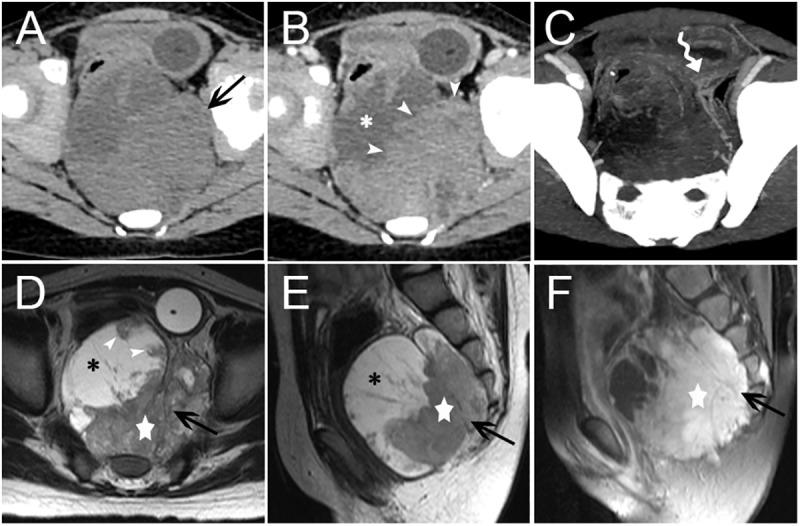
Presacral Region pPNET in a 5-year-old Female (Case no. 3). CT images showed an irregular, mixed solid cystic mass (arrow) with an indistinct margin in the presacral region (A). The necrotic or cystic portion (asterisk) did not show clear enhancement, but the solid portion (arrowheads) presented with intermediate heterogeneous enhancement (B). Blood supply arteries could be observed (curved tail arrow) (C). Non-contrast MRI images showed the solid components (five-pointed star) of the mass (arrow) was isointense on T1WI images and mildly, heterogeneous hyper-intense on T2WI images. The lateral necrotic or cystic portion (asterisk) showed hypo-intensity on T1WI images and heterogeneous hyper-intensity on T2WI images. Mural nodules were observed (arrowhead) (D-F). On contrast-enhanced MRI images, the solid components (five-pointed star) of the mass showed marked heterogeneous enhancement, with low signal separation lines within the tumor (F).
